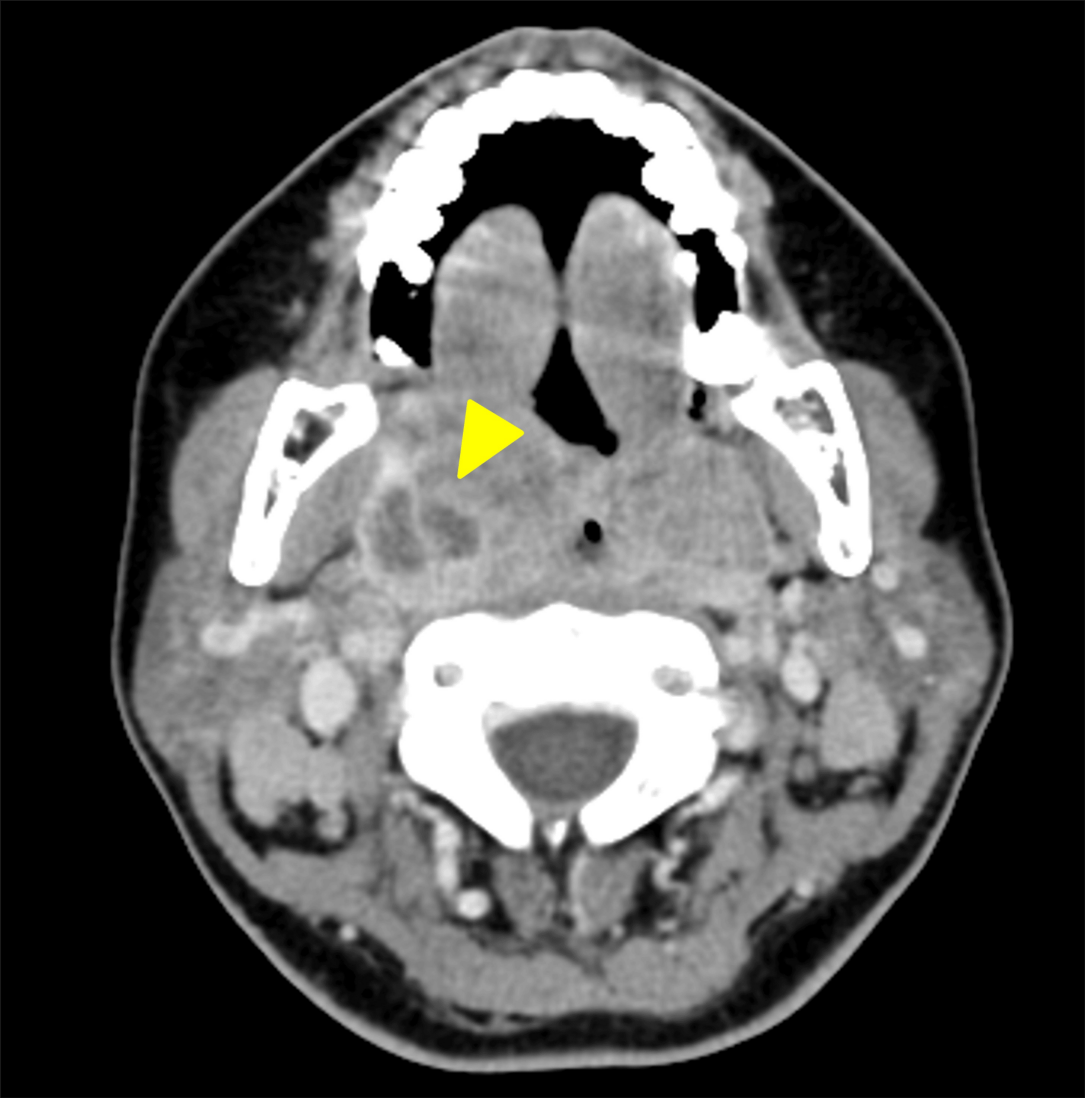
Contrast-enhanced CT on hospital day 3 Axial neck image demonstrating a right peritonsillar abscess (arrow head) with a 13-mm hypodense lesion adjacent to the right tonsil. CT: computed tomography
